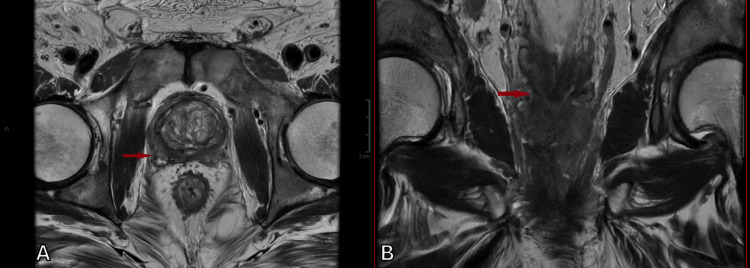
Extraprostatic involvement from prostate cancer (indicated by the red arrow) arising from the right peripheral zone, with bulging of the prostatic capsule and superior extension into and invasion of the right seminal vesicle, as seen in axial and coronal T2WI (A) Axial T2WI. (B) Coronal T2WI. T2WI, T2-weighted imaging
